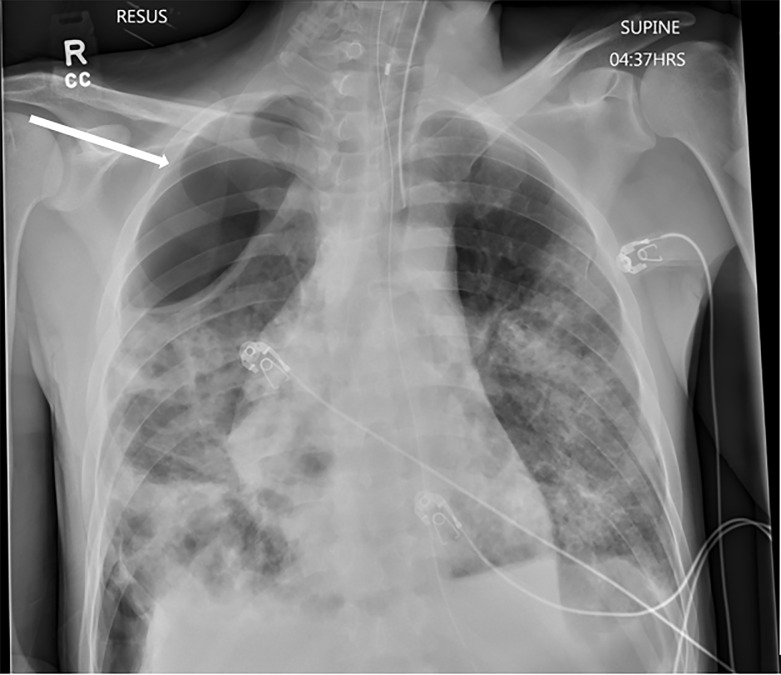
Post-intubation chest anterior-posterior radiograph demonstrating pneumonia and gigantic bulla (white arrow).
